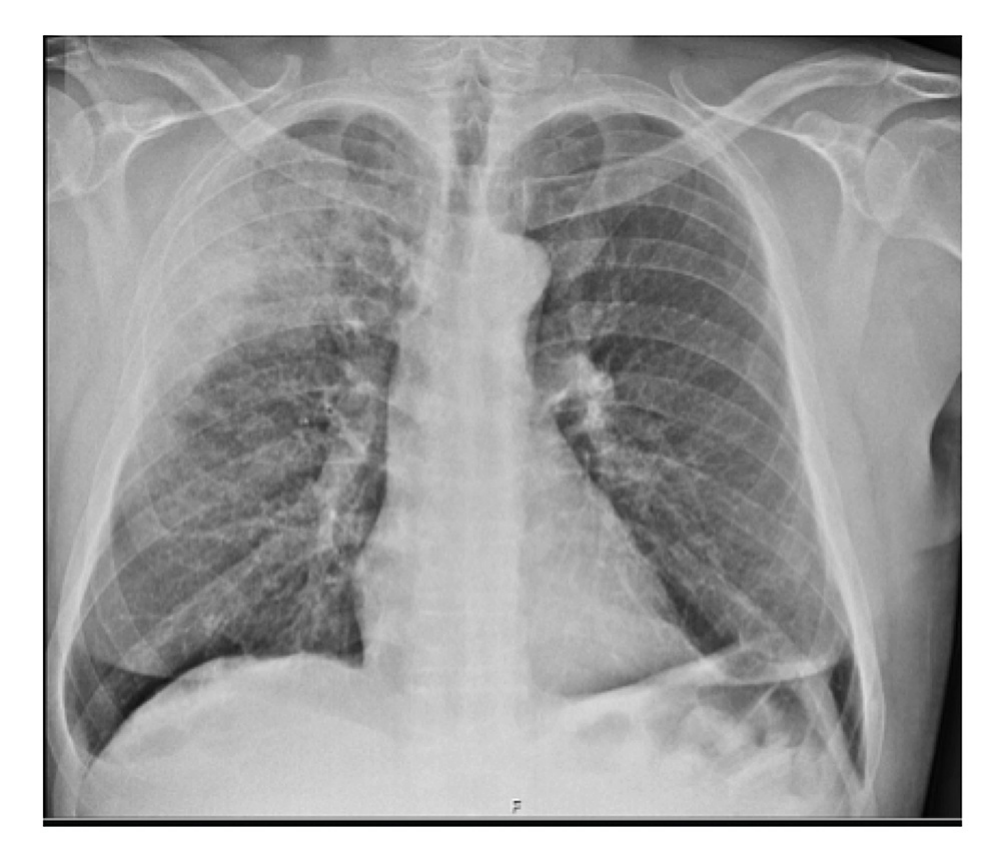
Anteroposterior chest radiography.
